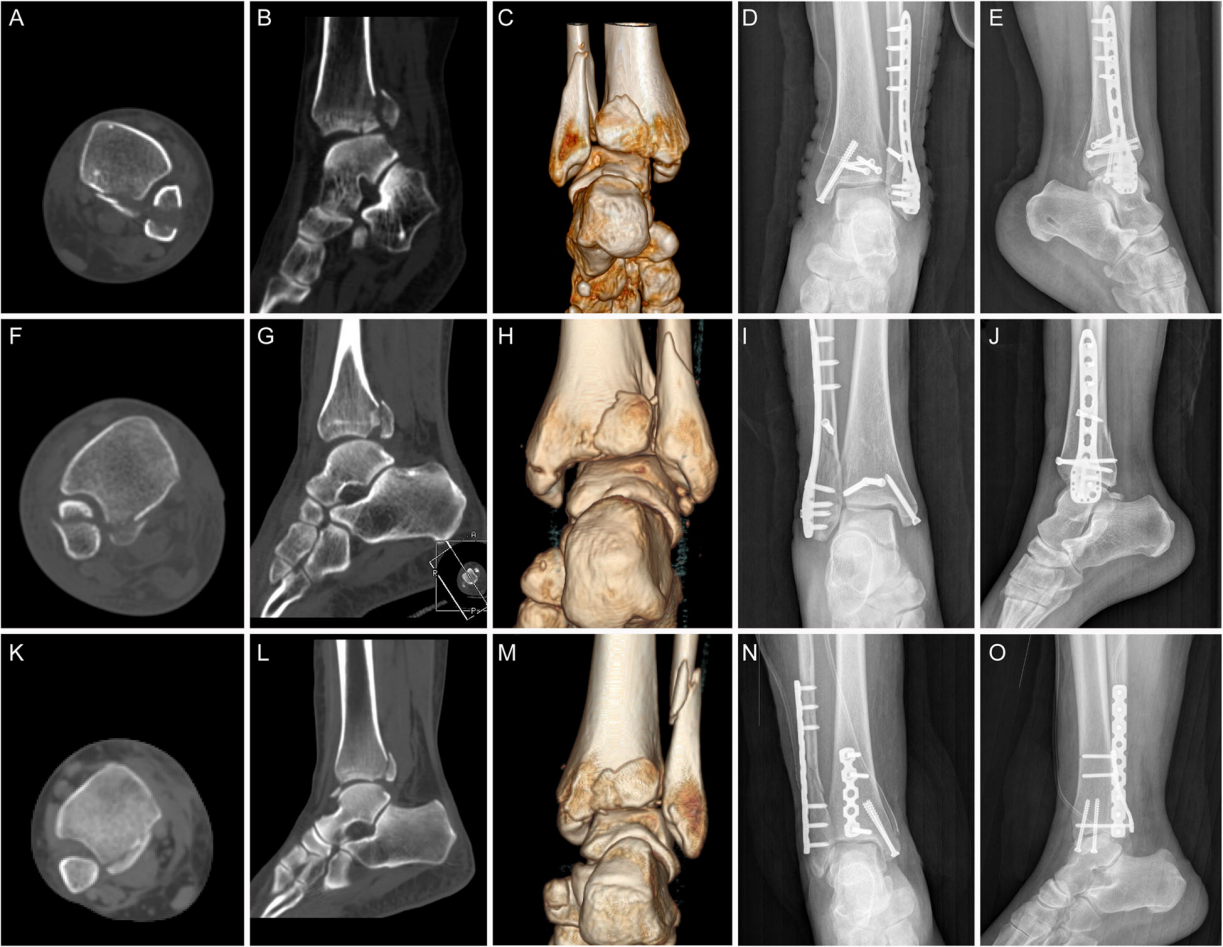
CT and X-ray images of patients who had posterior malleolar fracture with fragment size of < 15%. a, b, c, f, g, h, k, l, m: preoperative CT images; d, e: Postoperative X-ray of a patient showing fixation with a PA screw; i, j: postoperative X-ray of a patient showing fixation with a AP screw; n, o: postoperative X-ray of a patient showing fixation with a posterior plate
